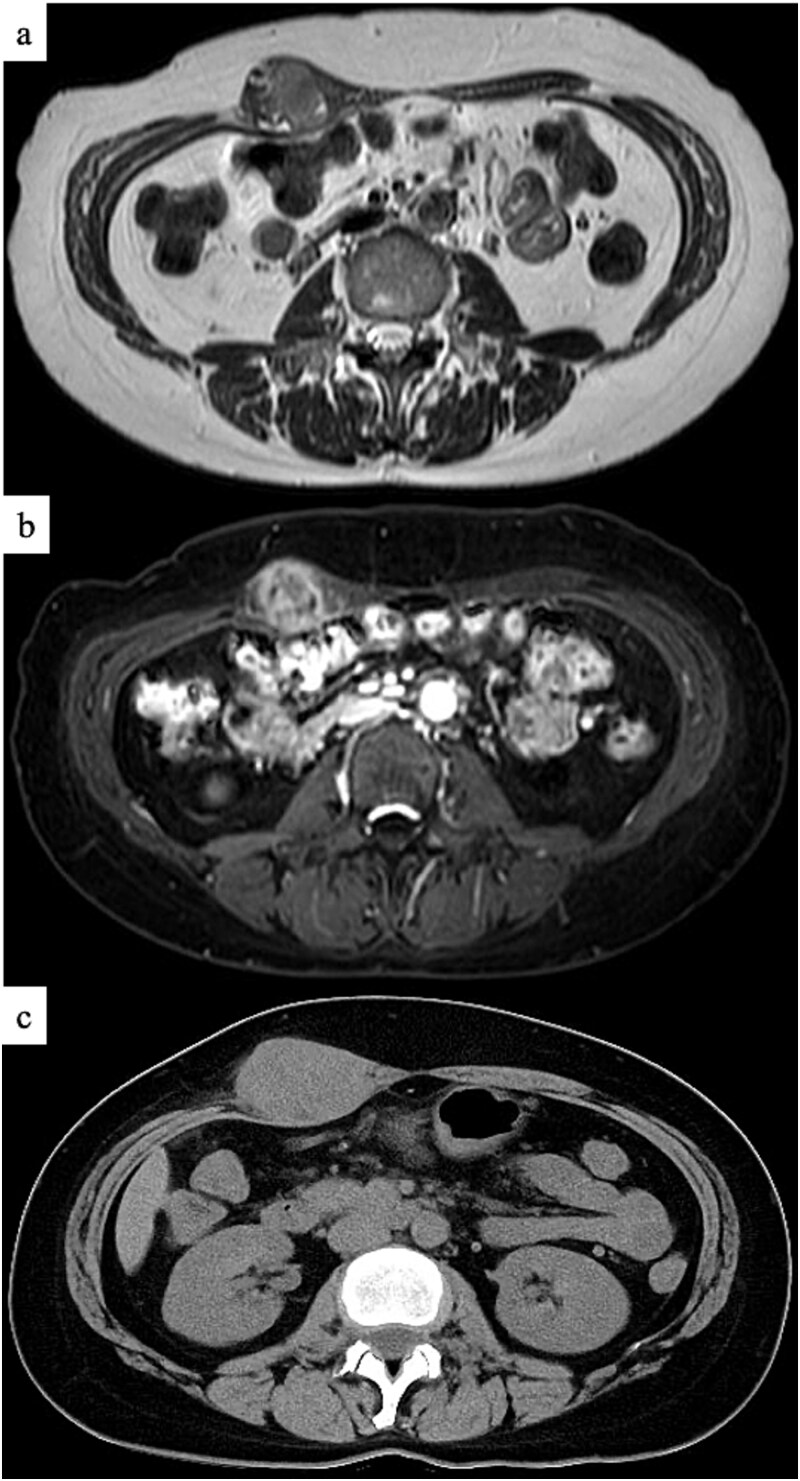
MR images showing a mass in the right abdominal wall. (a) T2WI, (b) Gadolinium (Gd) enhanced. (c) CT image showing a homogeneous isodense lesion without calcification on the right abdominal wall.
